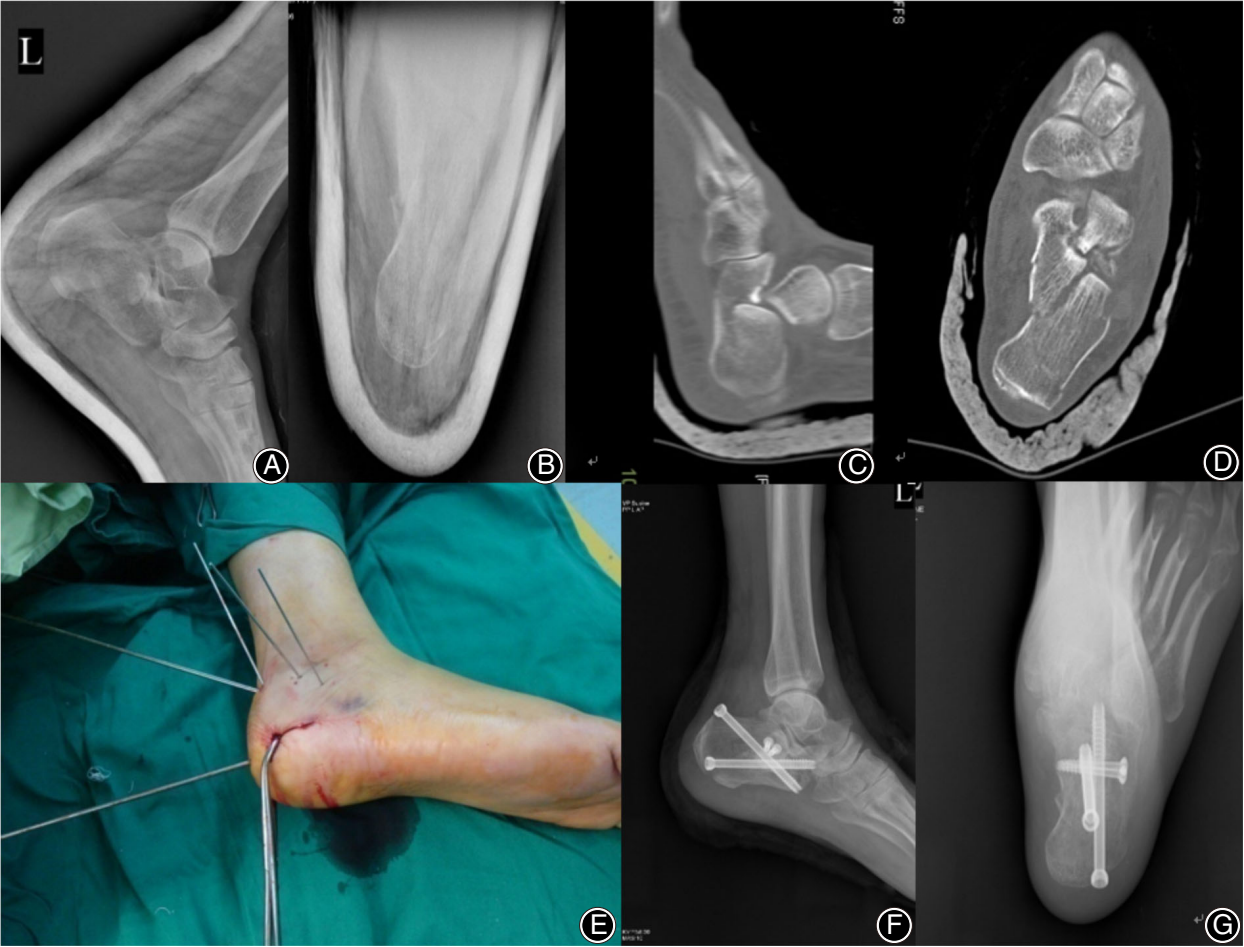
A 58‐year‐old female patient fell from height, resulting in an intra‐articular calcaneal fracture. (A) Preoperative lateral X‐ray and (B) preoperative axial X‐ray. CT image (C, D): A Sander type II calcaneal fracture with a subtalar articular surface collapse. (E) Intraoperative incision picture. (F) Postoperative lateral X‐ray shows a good reduction of calcaneal length, height, Bohler's angle, and Gissane's angle. (G) Postoperative axial X‐ray shows a good reduction of calcaneal width.
